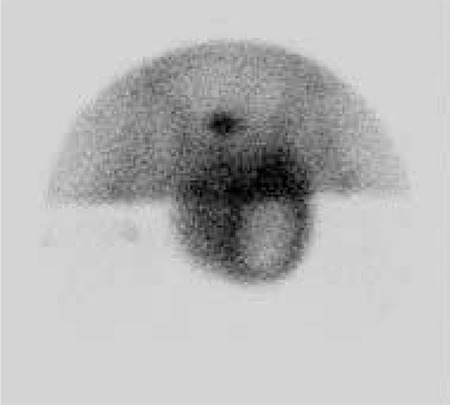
The anterior pinhole view of testis scintigraphy showing hypoactive left testicular area surrounded by an hyperactive halo.
